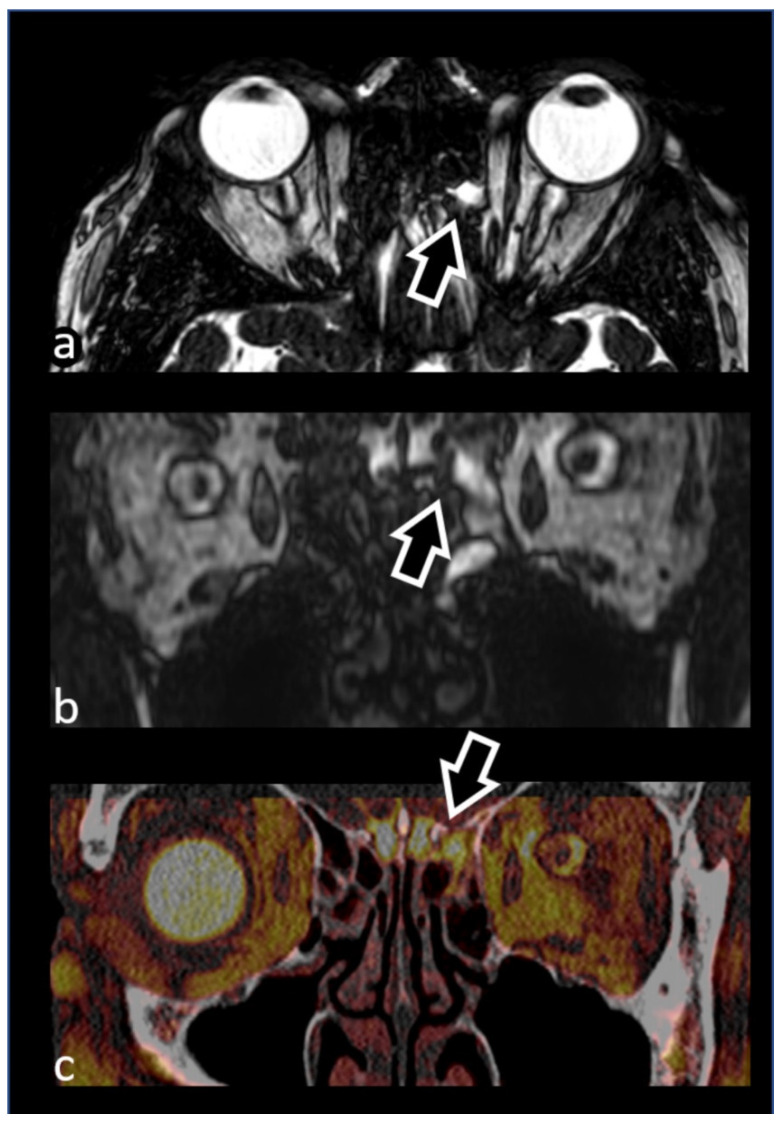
74-year-old female patient presenting with watery fluid leaking from her left nostril, suspected for cerebrospinal fluid (CSF) leakage. Axial (a) and coronal (b) 3D CISS images show a CSF-like linear hyperintensity extending from a subarachnoid space in the left frontal pole into an anterior ipsilateral ethmoid cell (arrows). At that level, computed tomography (CT) performed on the same day revealed a bony defect of the cribriform plate. These findings, well represented on postprocessed fused CT and MR-cisternographic images (c), were in keeping with a CSF fistula. A left partial ethmoidectomy was performed the following week, revealing a focal meningocele and confirming the CSF fistula. The meningocele was repaired, with a complete resolution of symptoms at one-month follow-up.
